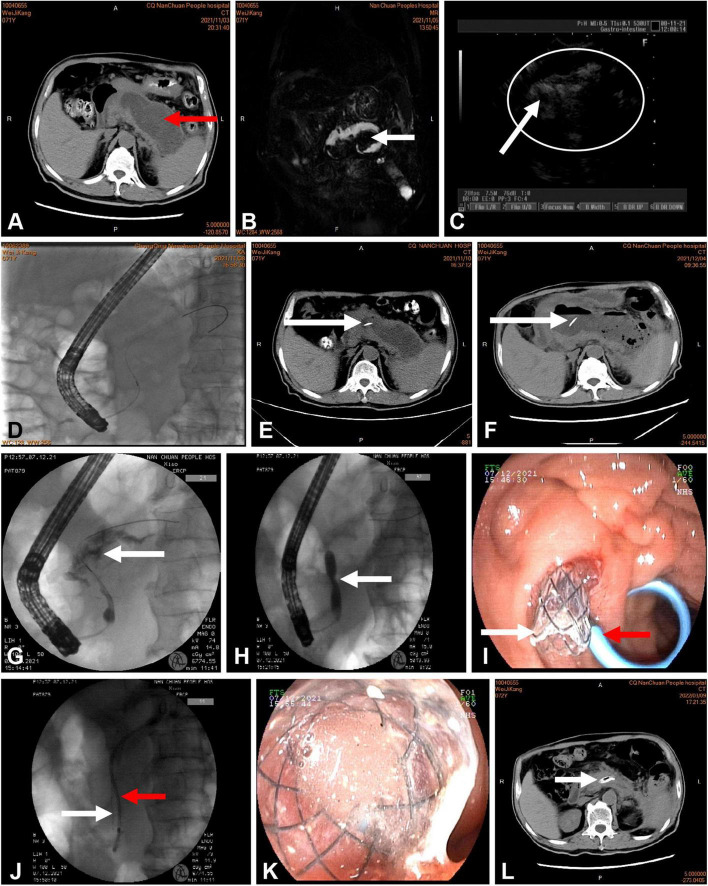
(A) Computed tomography (CT) of the abdomen showing more than 50% pancreatic parenchymal necrosis and pancreatic fluid collection 102 mm in diameter (red arrow). (B,C) Rich debris was present in the cavity, as shown by magnetic resonance cholangiopancreatography (MRCP) (white arrow) and endoscopic ultrasound (white circle). (D) Endoscopic retrograde cholangiopancreatography (ERCP) showing a disrupted pancreatic duct communicating with the WOPN. (E,F) CT showing a plastic stent (white arrow) communicating with the WOPN. (F) The cavity was not reduced at 4 weeks after intervention. (G) ERCP showing extravasation of contrast material (white arrow) from the region of the pancreatic neck. (H) Balloon dilation of the pancreatic duct stenosis (white arrow). (I,J) Deployment of a metal stent (white arrow) and a plastic pancreatic stent (red arrow) inside the WOPN. (K) Drainage of necrotic tissue and fluid from the metallic stent. (L) CT performed 3 months after the end of active drainage. In the main pancreatic duct, the transpapillary metal and plastic pancreatic stents were visible.
